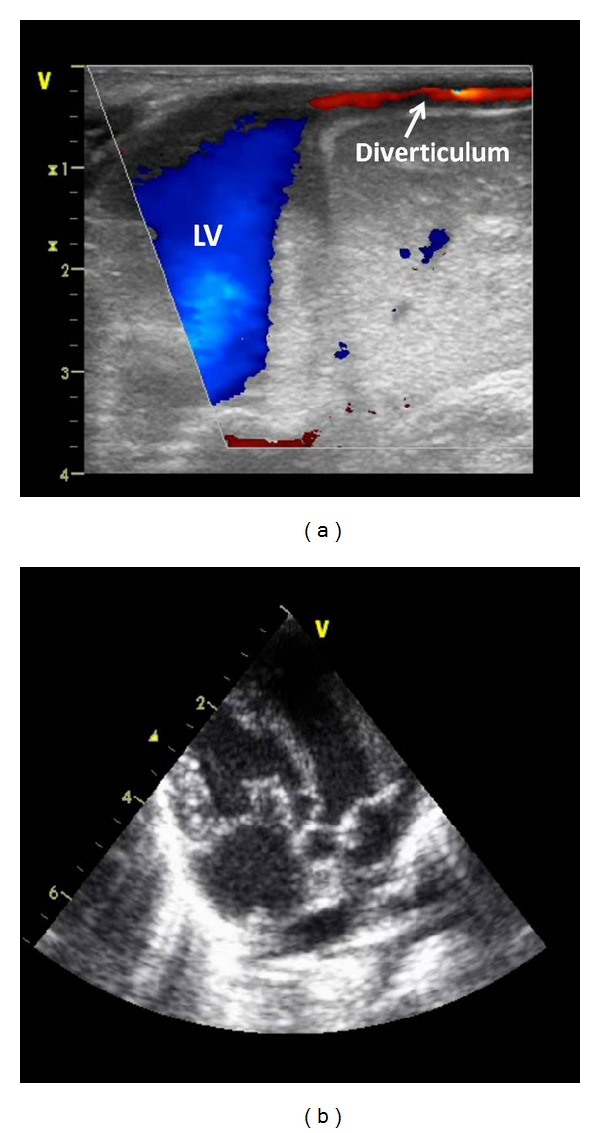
The echocardiography demonstrated blood flow in and out of the pulsatile mass, a left ventricle diverticulum, dextrorotation ofthe heart, a triatrial situs solitus, a small ventricular septal defect, and normal venous anatomy were demonstrated.
